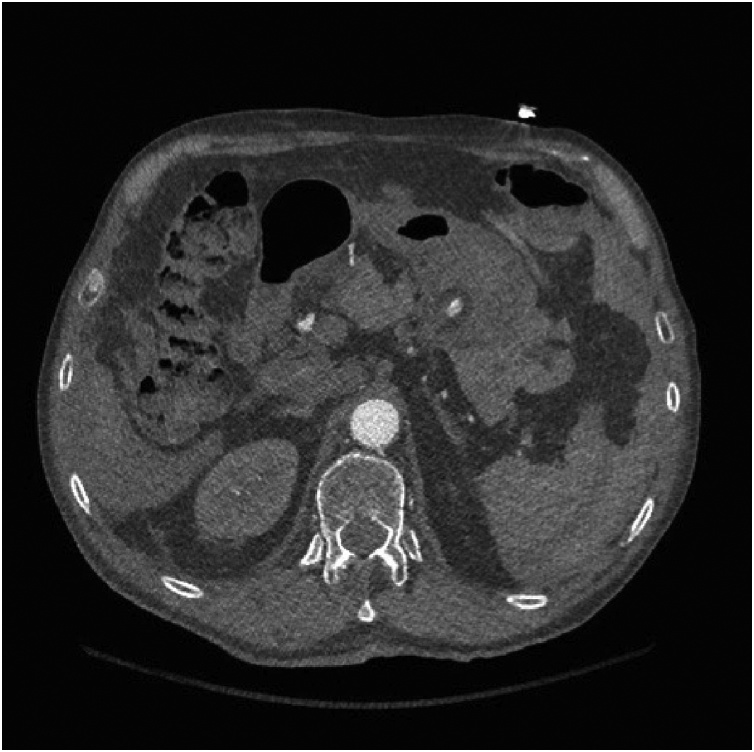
CT Angiogram in the arterial phase demonstrating a 9 mm focal area of increased attenuation along the lesser curvature of the stomach representing a small pseudoaneurysm of the left gastric artery, with no active extravasation appreciated.
